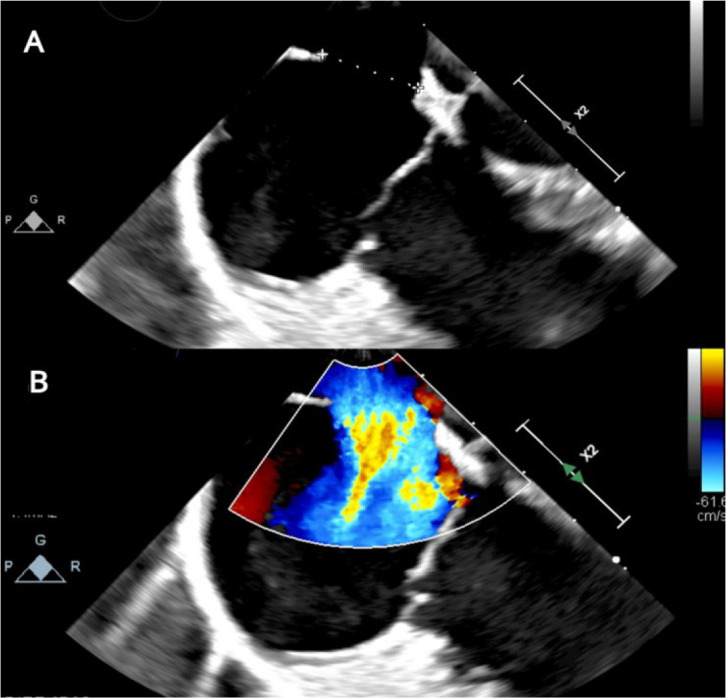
Apical four-chamber transesophageal echocardiogram, two-dimensional
